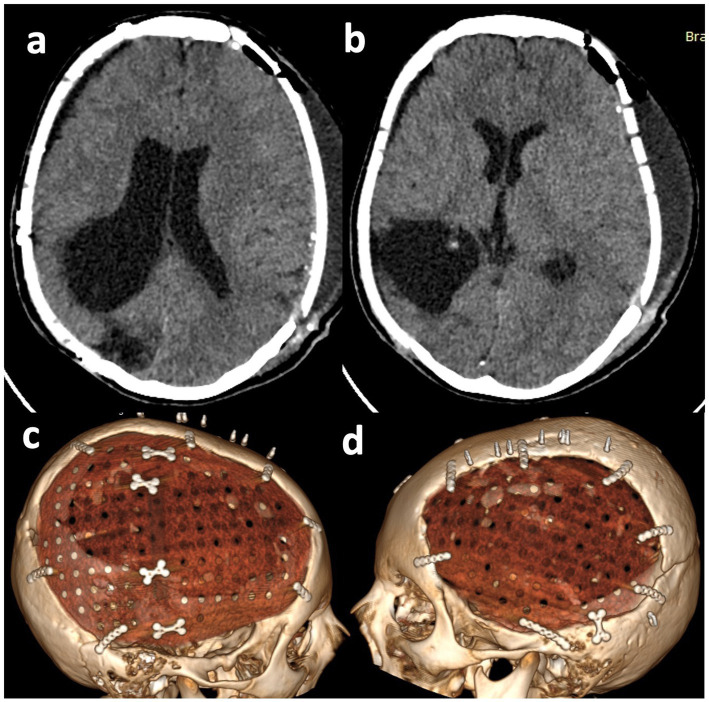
Head CT images (a,b) of a patient with severe traumatic brain injury, who underwent restorative cranioplasty following decompressive craniectomy. 3-D reconstruction of postoperative head CT scan (c,d) of the same patient showing right-side (c) and left-side (d) restoration.
